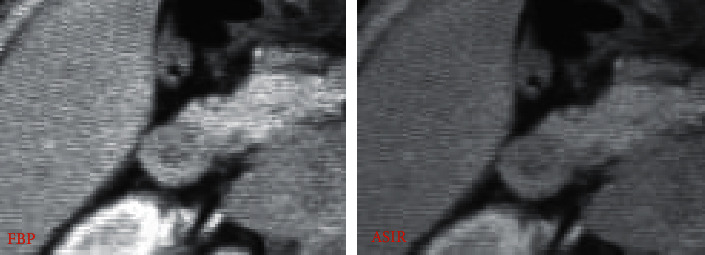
The partially enlarged views of gallbladder imaging after reconstruction of the abdominal CT image of an ICP patient in two ways. *Note.* The average scores of subjective evaluations of FBP reconstructed images show image quality of 2.38 points, noise of 2.29 points, and artifacts of 4.49 points; the average scores of subjective evaluations of ASIR reconstructed images show image quality of 3.94 points, noise of 1.81 points, and artifacts of 2.38 points.
